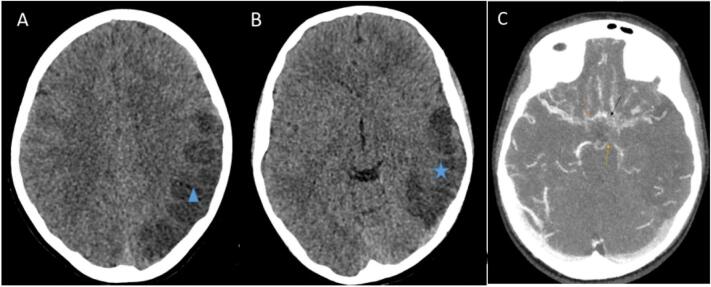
Selected axial brain CT scan images were done for the same patient, Brain CT scan without contrast (A + B) and brain CT angiography with MIP (maximum intensity projection) technique. They are showing left parieto-occipital (blue triangle in an A image) and temporal (blue star in B image) cortical and subcortical low attenuating area denoting subacute ischemic injury in the territory of left MCA (middle cerebral artery) and left PCA (posterior cerebral artery). (C) Image shows a complete cutoff at M1 segment of the left middle cerebral artery (black arrow) and narrowing at M1 segment of the right MCA (orange arrow) and left PCA (yellow arrow).
